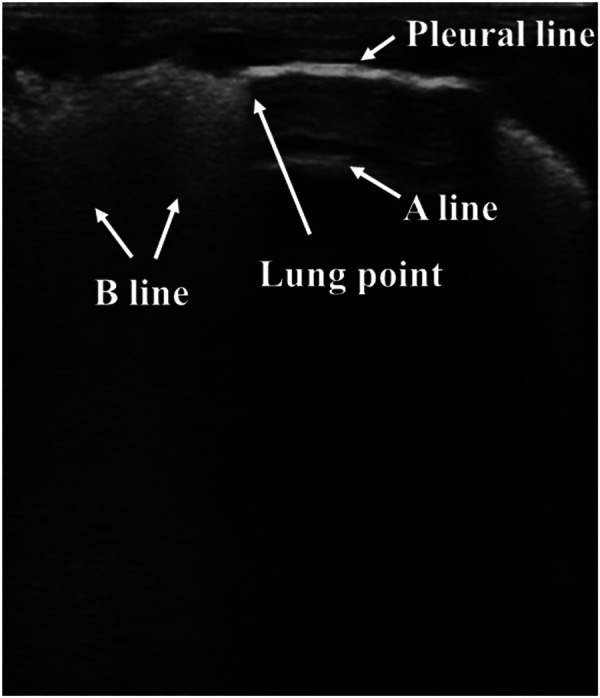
Pneumothorax on lung ultrasonography. Lung point: the transition from the B-lines area (left side) to a hypoechoic area (right side).
